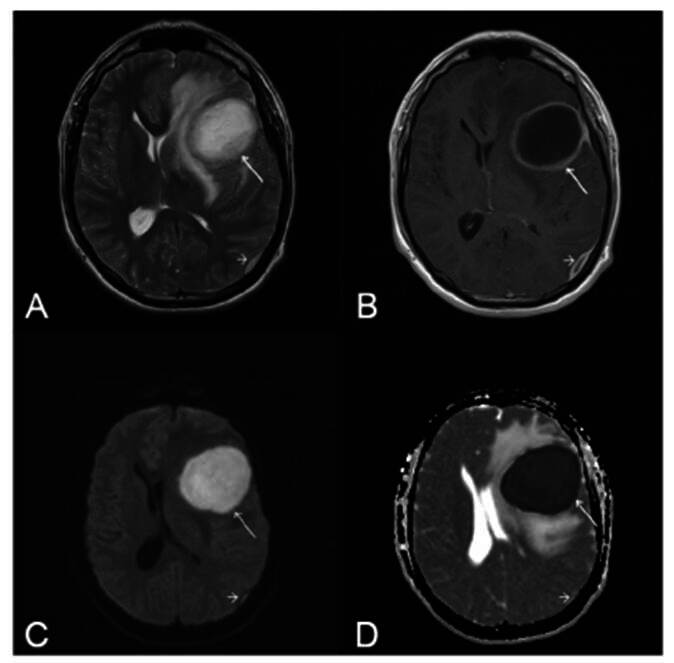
Axial T2 weighted image (A). T1 weighted image after contrast administration (B). Diffusion-weighted imaging (DWI) (C). Apparent diffusion coefficient (ADC) map (D). These images show an expansible intracranial lesion in the left frontal lobe, with peripheral ring enhancement and perilesional edema (arrow) (B), and restricted diffusion characterized by hyperintensity on DWI (arrow) (C) and hypointensity on the ADC map (arrow) (D), as well as ipsilateral parieto-occipital epidural empyema (short arrows in A, B, C, and D).
